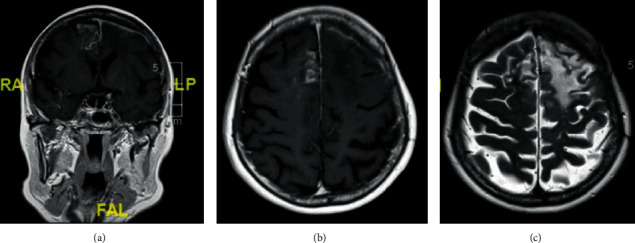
MRI brain. (a, b) Coronal and axial T1 postcontrast images showing pachymeningeal enhancement in the left frontal region and leptomeningeal enhancement in the left superior and middle frontal sulci, with vasogenic oedema. A nodular leptomeningeal enhancement is noted within the right parafalcine and superior frontal sulci with minimal underlying vasogenic oedema. There was no diffusion restriction. (c) Axial FLAIR image showing the extent of the vasogenic oedema.
